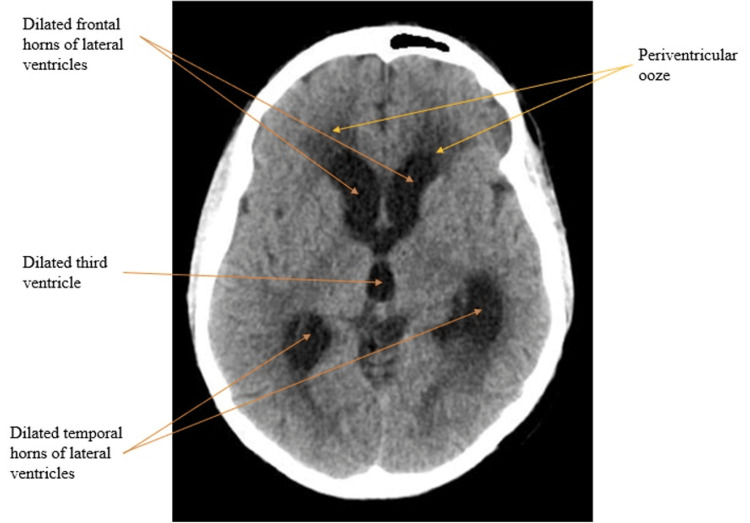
Axial NCCT scan Axial NCCT scan of the brain showing dilated frontal and temporal horns of the lateral ventricle and dilated third ventricle along with periventricular ooze suggestive of hydrocephalus in a TBM patient. NCCT: non-contrast computed tomography; TBM: tuberculous meningitis
